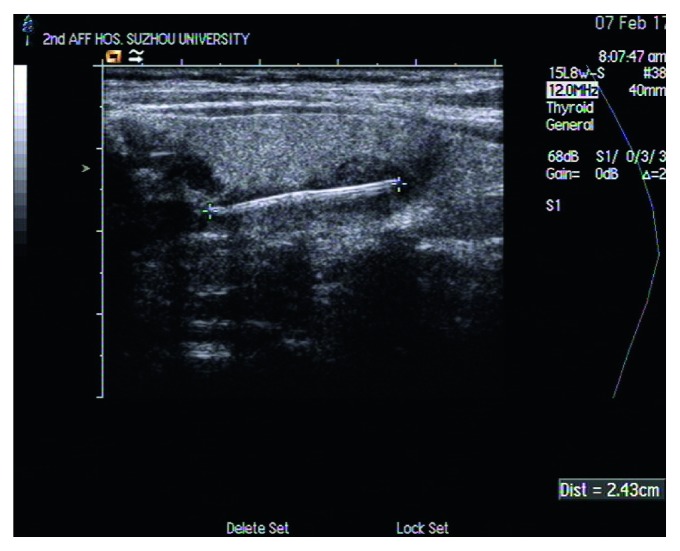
The features of ultrasonography. The presence of a foreign body that was approximately 2.43 cm hyperechoic linear image embedded in the left part of the thyroid lobe, and part of it was located outside the back membrane of the thyroid. A hypoechoci area surrounding the foreign body is regarded as the ultrasonic characteristics of inflammatory response.
